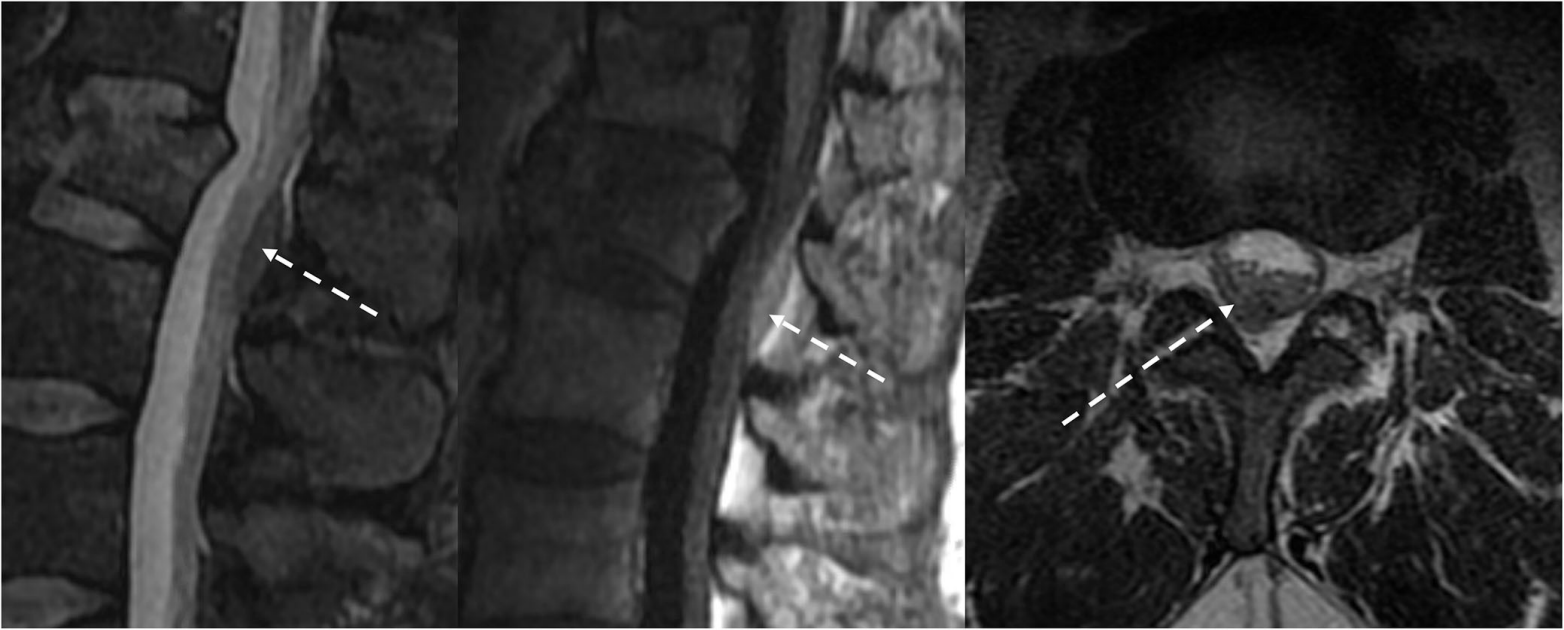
(a,b) MRI of the lumbar spine in this patient with traumatic injury demonstrated an L1 burst compression fracture and a small dorsal epidural hematoma at the L1-2 level. (C) The T1 hyperintense and T2 hypointense biconvex-shaped epidural hematoma effaces the epidural fat.
